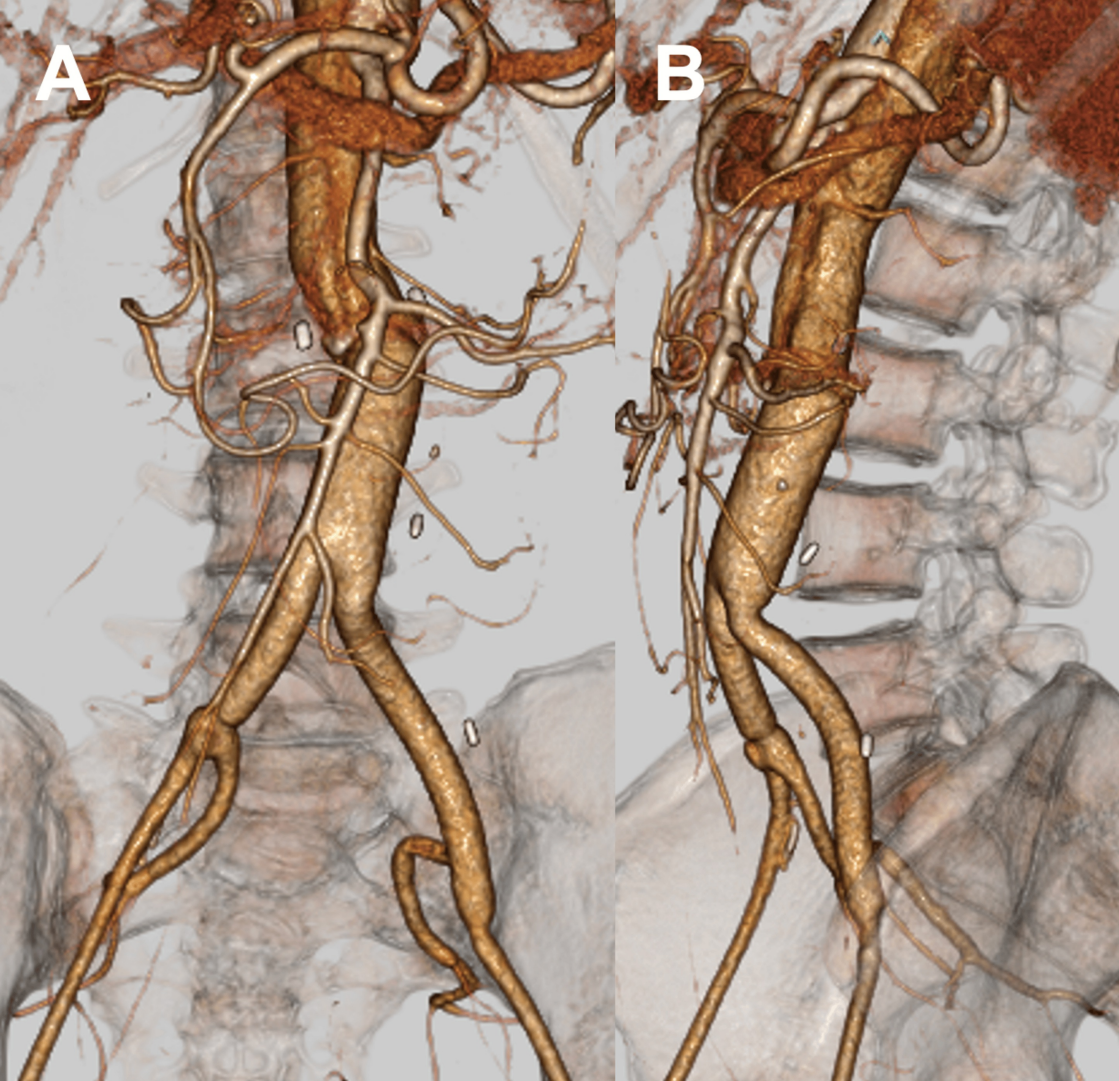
3D CT images after Y-graft replacement with a double-barrel anastomosis technique A. 3D CT image in the frontal view. B. 3D CT image in the left anterior oblique (LAO) view CT: computed tomography
